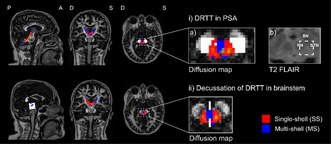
FIGURE 1i, SS and MS DRTT projections within the PSA (white box), shown on diffusion image (a) and T2‐FLAIR (b), highlighting the subthalamic nucleus (STN), substantia nigra (SN) and red nucleus (RN). ii, Decussating fibres crossing the brainstem midline.
